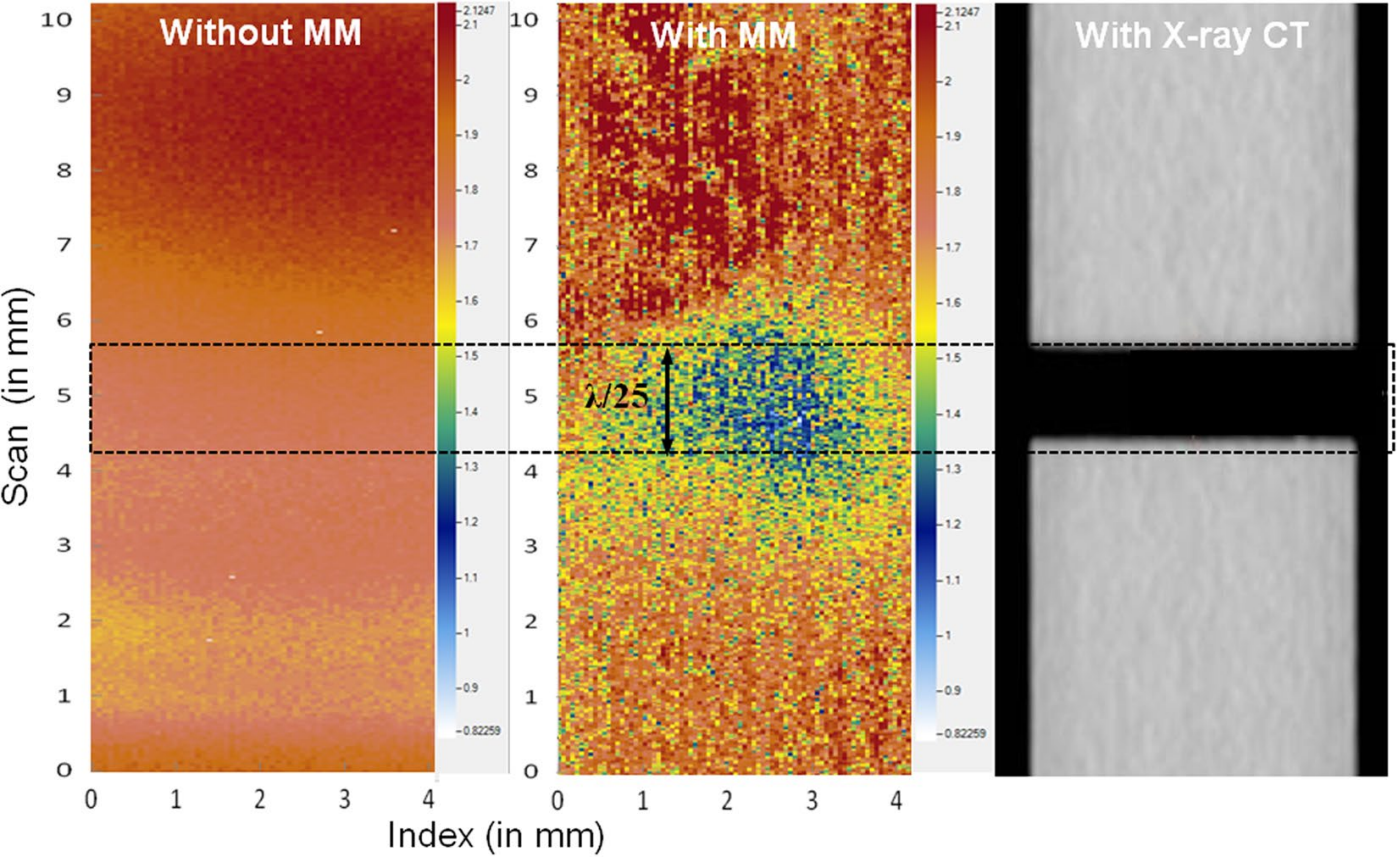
Image obtained by ultrasonic immersion C-scan without and with metamaterial lens, in comparison with that from X-ray μCT scan.
